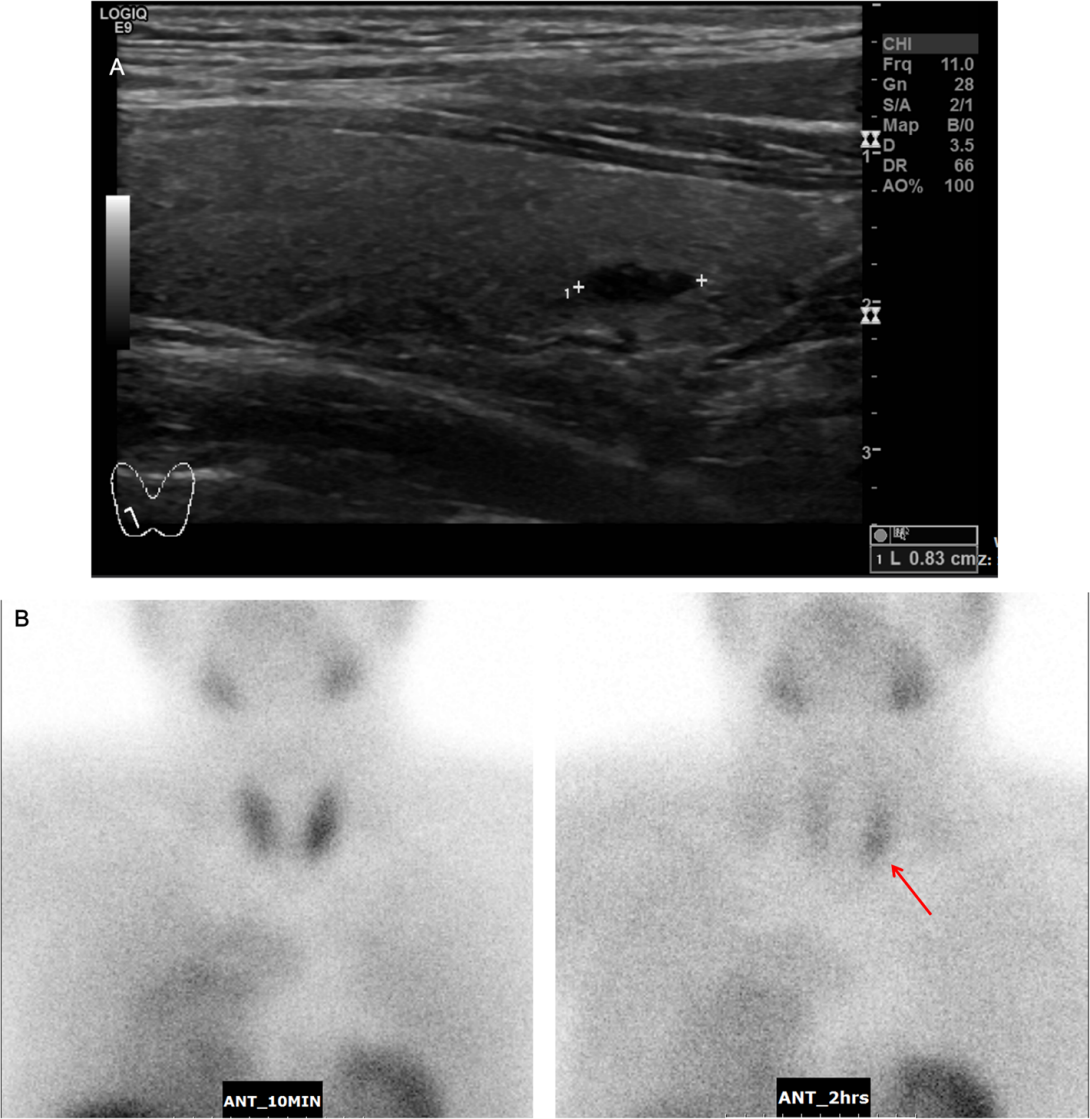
Images of the parathyroid glands. a The longitudinal scan of neck ultrasound demonstrates a well-defined hypoechoic solid mass posteroinferior to the left lobe of the thyroid gland. b Early and late 99 m Tc-sestamibi scintigraphy parathyroid scan images of the neck and anterior mediastinum at 10 min and 2 h, showing increased focal uptake (red arrow) suggestive of left inferior parathyroid adenoma
